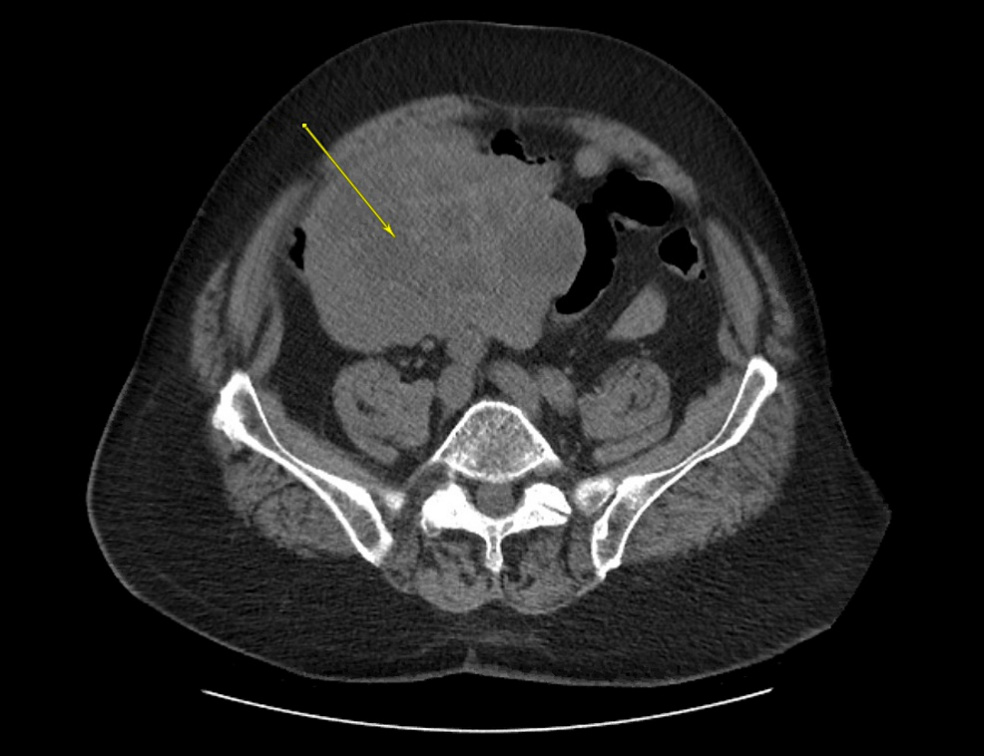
CT abdomen and pelvis The yellow arrow shows a lesion measuring 13.4 cm x 11.2 cm in the lower abdomen
